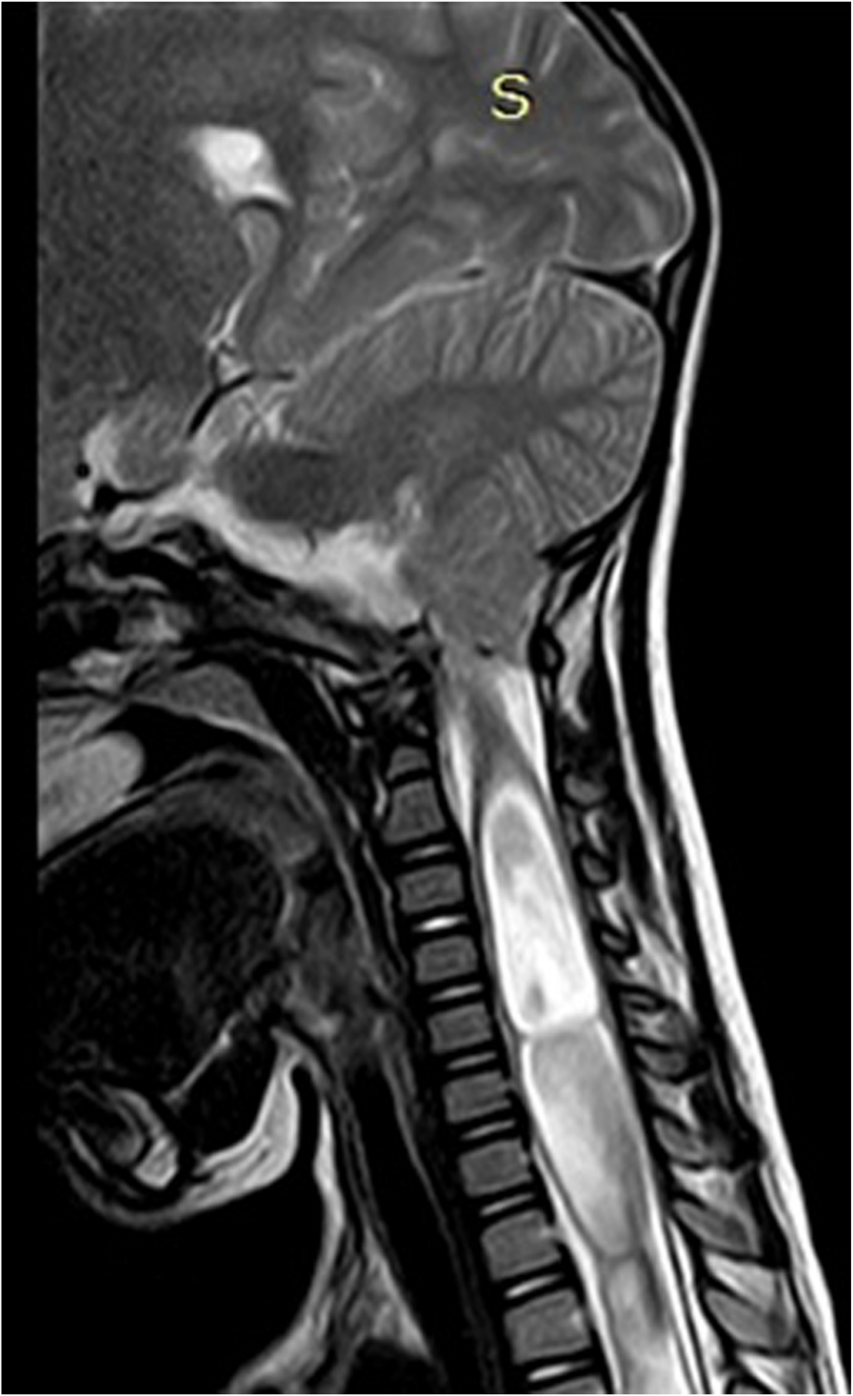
Sagittal T2-weighted MRI of cranio-vertebral junction and cervical spine showing tonsillar herniation and central cavitary lesion suggestive of Chiari malformation type 1 and syringomyelia
